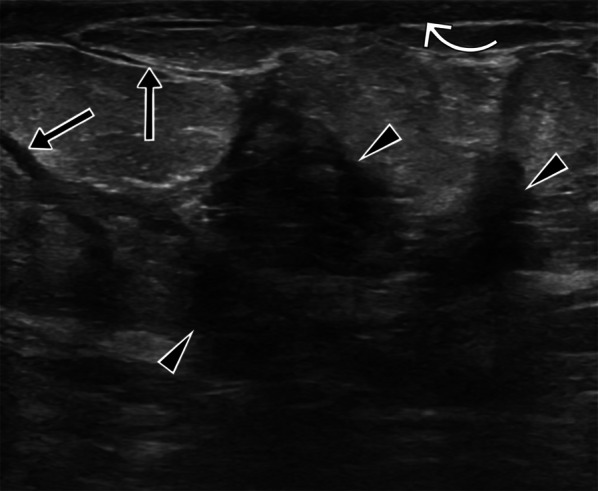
A 24-year-old and 30-weeks pregnant female patient. Ultrasound images show parenchymal edema in the right breast and abscesses with dense content (arrowheads), fistula tracts (arrows), and thickening of the skin (curved arrow). BIRADS 4
